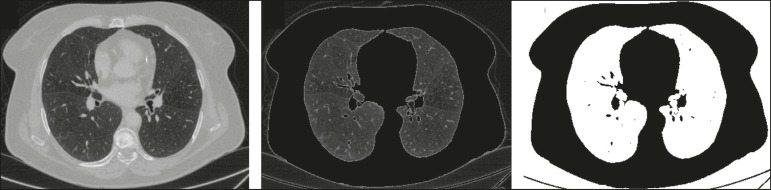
Semiautomated segmentation of the lung on a CT scan of the chest with 256 gray levels and a user-defined threshold of 115 Hounsfield units: the original CT image (image on the left) is thresholded (to detect the edges) and transformed into a binary image (to separate the lungs).
